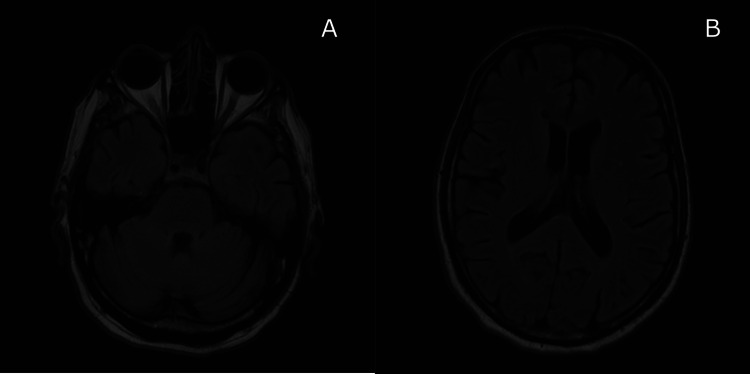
MRI of brain three months after the initial onset of symptoms revealed A) mild hyperintensity of both superior cerebellar peduncles on FLAIR imaging and B) a small punctate hyperintensity of the left inferior temporal white matter.
